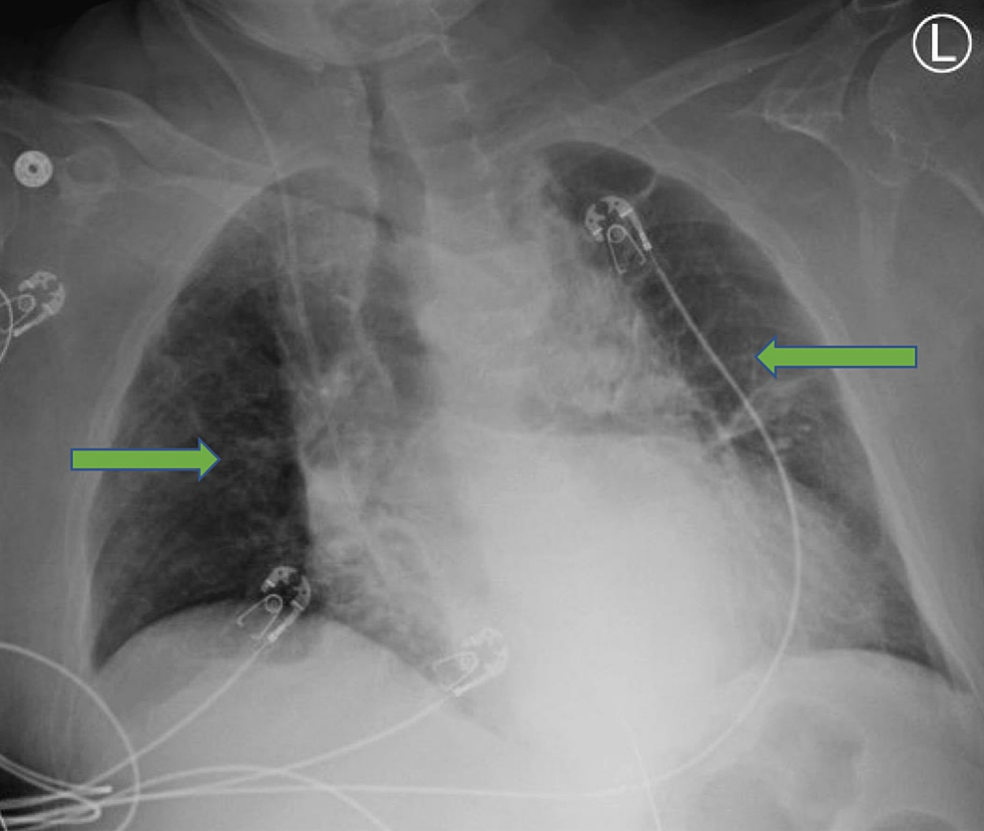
Chest X-ray after 5 days of cefiderocol treatment. Green arrows show reduced interstitial infiltrates after treatment.
